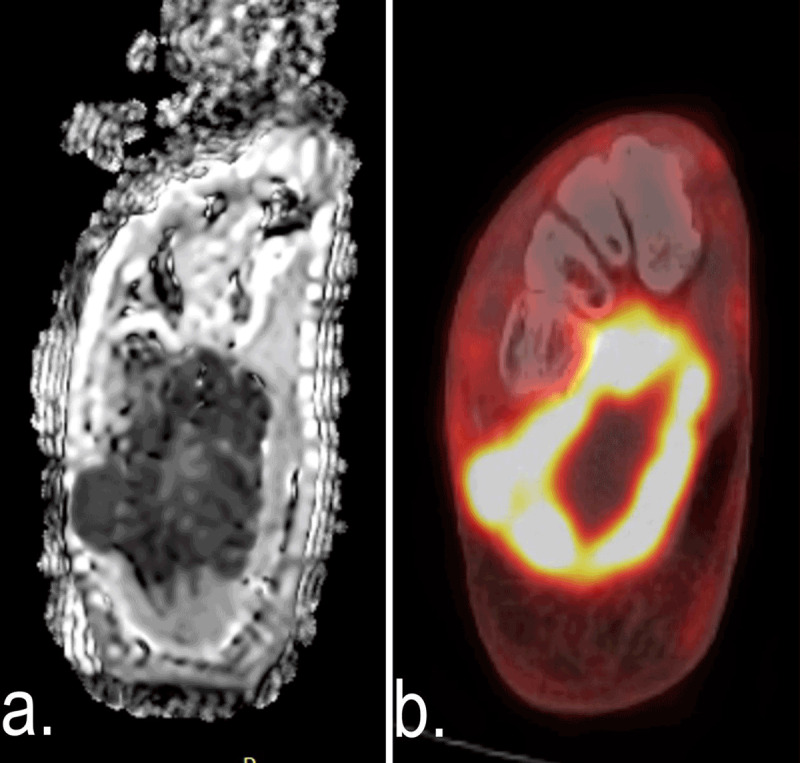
DWI (a) and FDG-PET/CT (b) images of a 35-year-old male with a soft tissue mass in the right foot. ADC_mean_, ADC_min_ and SUV_max_ of the lesion were 0.55 × 10^–3^mm^2^/s, 0.31 × 10^–3^mm^2^/s and 24.31, respectively. The lesion was diagnosed as undifferentiated pleomorphic sarcoma after core needle biopsy.
